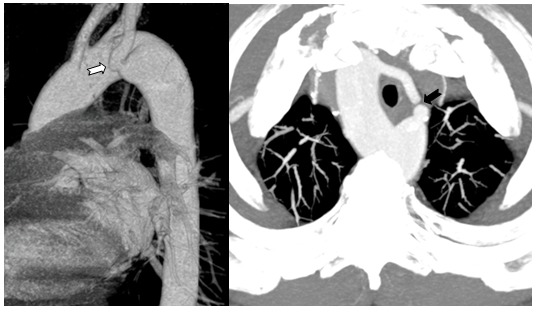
Thoracic CT. *On the left.* Volume rendering image for CT angiography of the thoracic aorta confirming that the left subclavian artery arises (white arrow) from a dilated segment of the distal arch (Kommerell’s diverticulum). *Right.* Maximum intensity projection (MIP) CT image of the upper thorax. Incomplete vascular ring for absence ligamentum arteriosum (black arrow). No signs of tracheal compression can be demonstrated.
